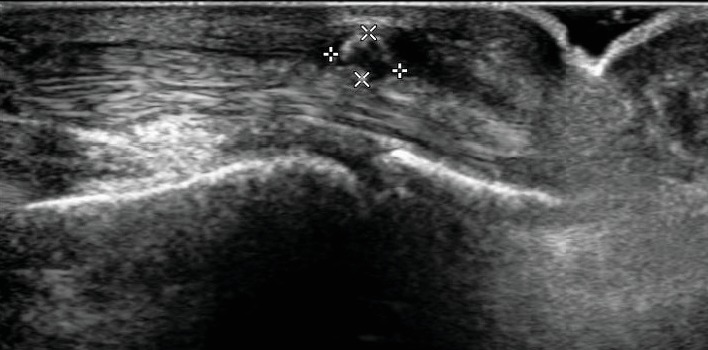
Ultrasound examination of the fourth finger of her left hand shows an avascular mass of about 6 × 2 mm, attached to the flexor tendons, anechogenic with an echogenic core.
